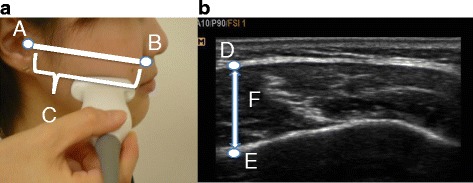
Masseter muscle thickness measurements. a Position of the probe during measurement. b Masseter muscle imaging with an ultrasonic diagnostic equipment. Upper edge of the tragus (B) Point under the nasal wing (C) Camper’s plane (D) Masseter muscle surface (E) Mandibular ramus (F) Masseter muscle thickness
